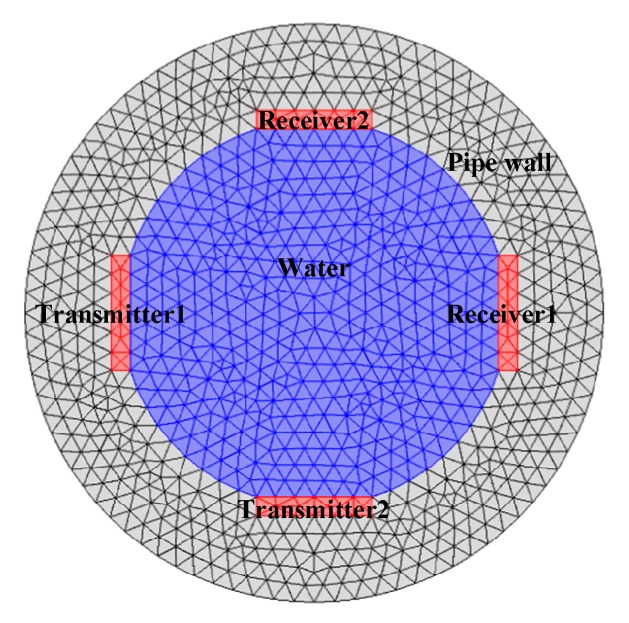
The two-dimensional meshed models of a mutually perpendicular ultrasonic sensor (MPUS).
